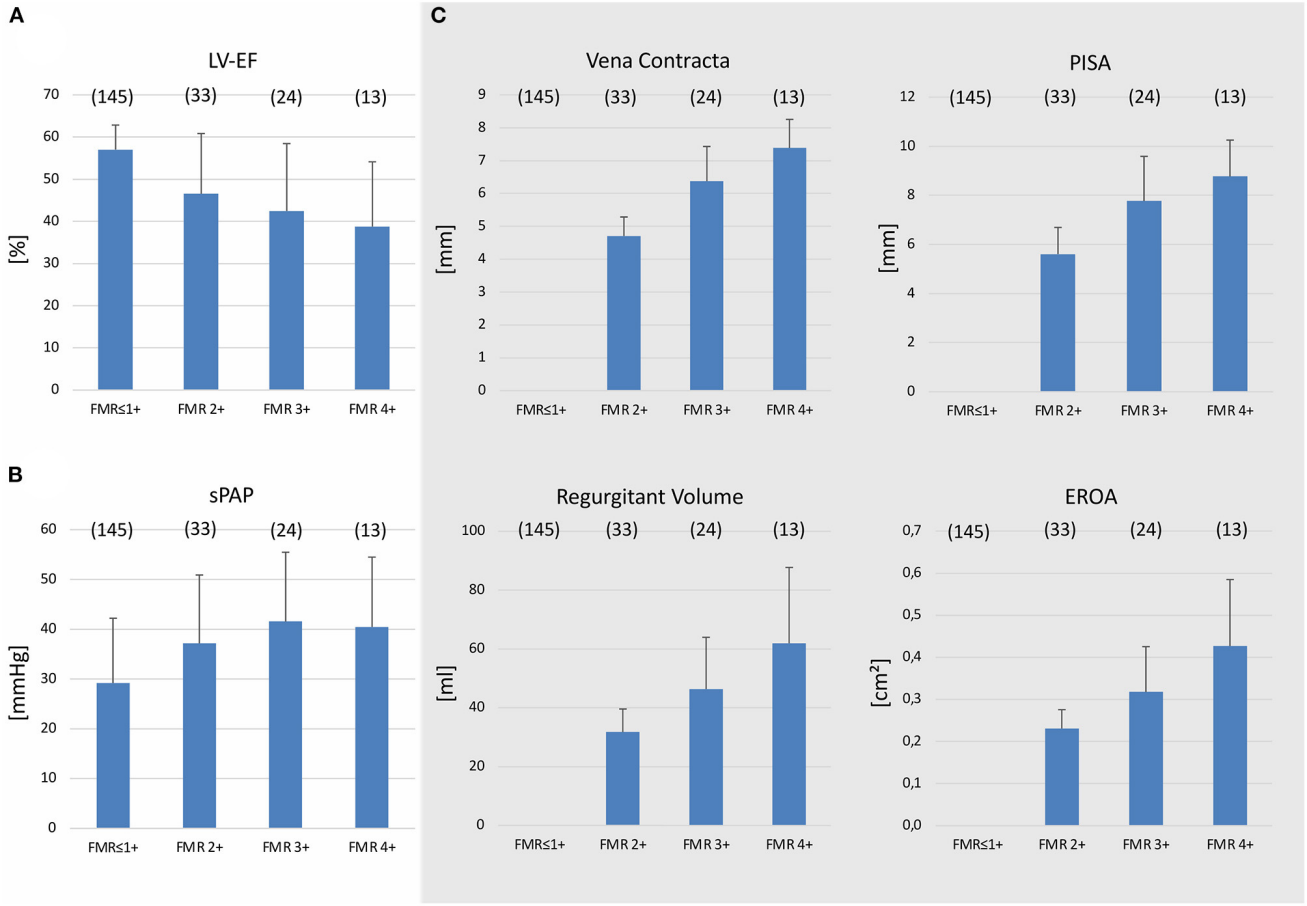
Echocardiographic evaluation of patients with and without functional mitral valve regurgitation. Left ventricular ejection fraction (LVEF) (A) and systolic pulmonary artery pressure (sPAP) (B) in patients with functional mitral regurgitation (FMR) ≥ 2+ or FMR ≤ 1+. Patients with FMR ≥ 2+ were separated according to a standard classification (FMR 2+, 3+, and 4+). (C) Quantitative echocardiographic assessment including measurement of vena contracta, proximal isovelocity surface area (PISA), effective regurgitant orifice area (EROA), and regurgitant volume in patients with FMR ≥ 2+. Mean ± *SD*.
